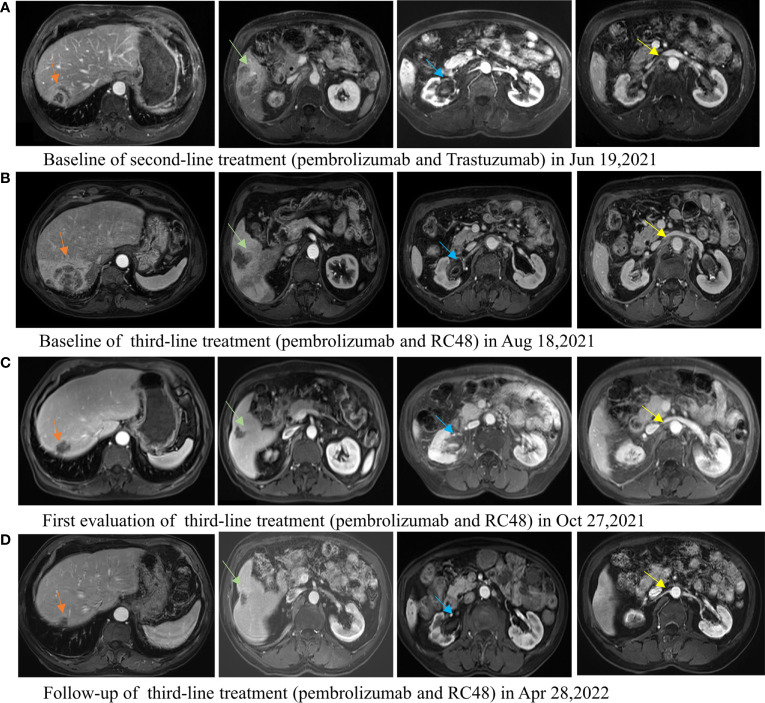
Lesions of the liver, ureteropelvic calyces, and lymph node change during the second-line treatment of pembrolizumab combined with trastuzumab and third-line treatment of pembrolizumab combined with RC48. (A) The baseline MRI image of the second-line treatment. (B) The image of tumor progression after second-line treatment, and the baseline image of the third-line treatment. The hepatic metastasis in the right posterior upper lobe was significantly enlarged and the other lesions reduced after second-line treatment. (C) The first efficacy evaluation after third-line treatment for 2 months: partial response. All tumor foci are reduced. (D) The image evaluation after third-line treatment for 8 months: partial response.
